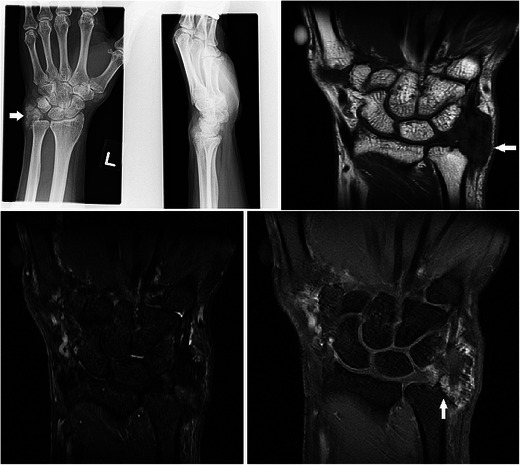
Gouty tophus in a 60-year-old male with known gout, presenting with a 6-month duration of gradual swelling along the ulna aspect of the left wrist. (a) Plain radiograph shows amorphous calcification adjacent to the ulna styloid (arrow). (b) T1w sequence shows an irregular lobulated mass of low signal (arrow). (c) The lesion was also hypointense on T2w-FS sequence. (d) Mild peripheral enhancement is seen, and there were also erosions of the underlying ulna styloid (arrow)
